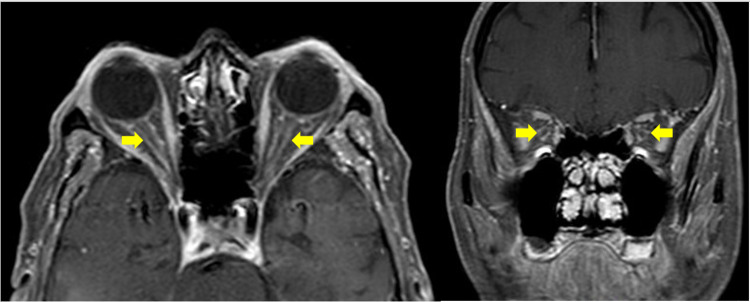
(Left) Axial view of gadolinium fat suppression T1-weighted MR image showed enhancement of bilateral optic nerve sheath “tram track sign” and (right) coronal view of gadolinium fat suppression T1-weighted MR image showed enhancement of bilateral optic nerve sheath “doughnut sign” at the intraorbital segment of optic nerve.
